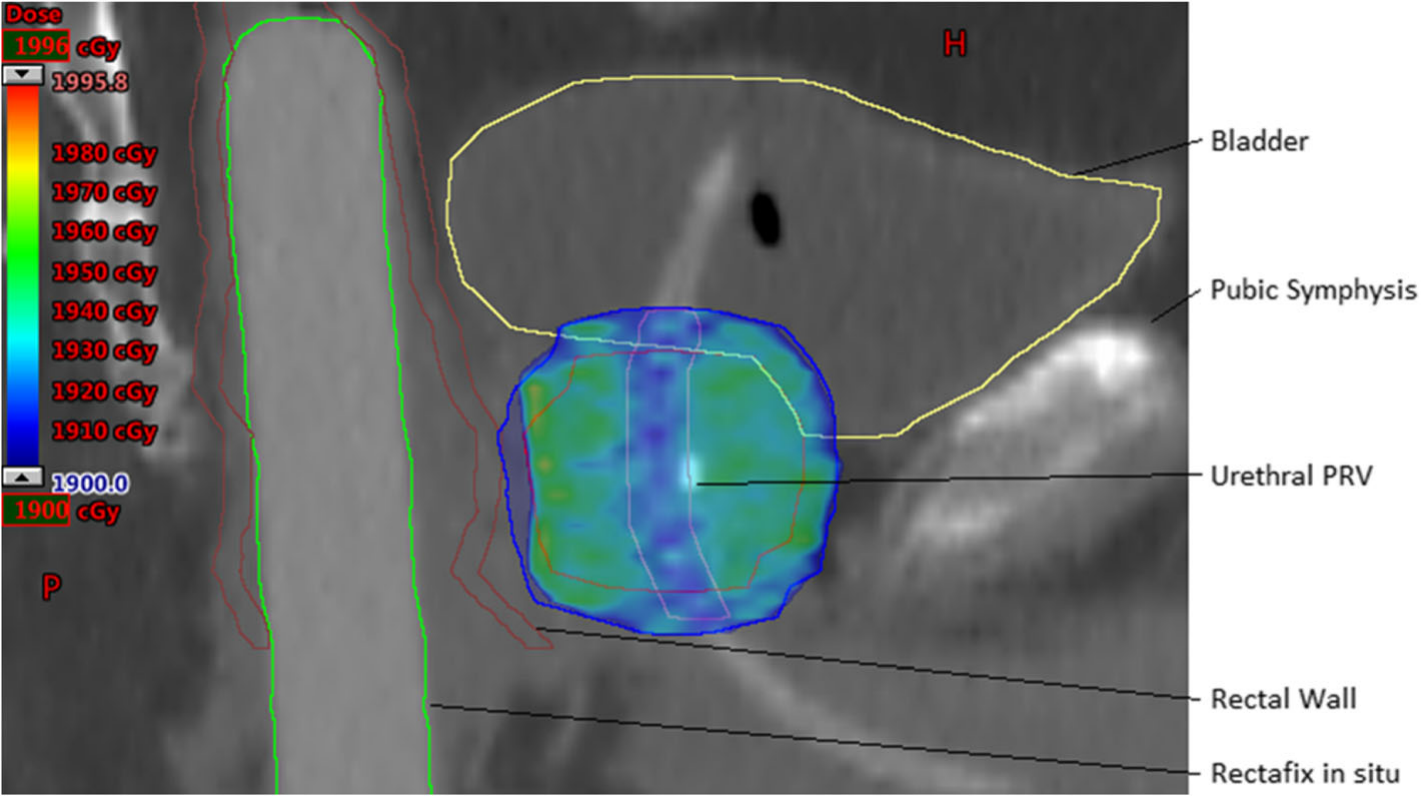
Sagittal CT view of example dose distribution. Note the high dose sparing of the Urethral PRV, and posterior PTVsbrt dose compromise to meet rectal dose constraints
